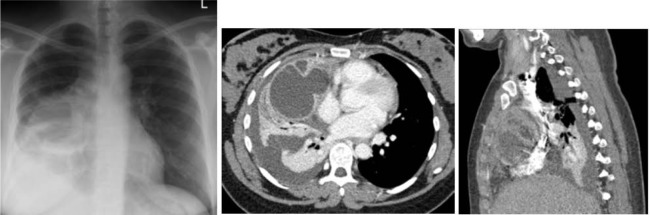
1) chest x-ray showing large mass in the right chest. 2) CT scan with cystic tumor in the anterior mediastinum displacing heart and lung
